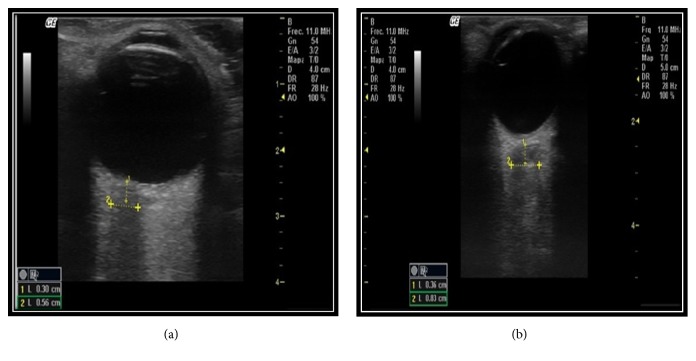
Ocular ultrasonography, mode B, with measurement of the optic nerve sheath, suggestive of endocranial hypertension, (a) transverse view, and (b) sagittal view.
